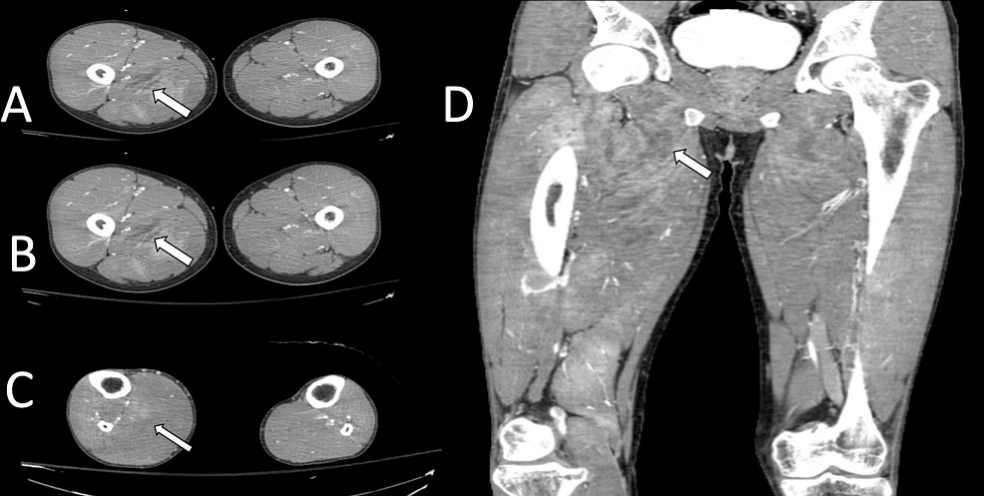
Axial and coronal computed tomography images of the lower extremities Axial and coronal computed tomography images of the lower extremities show extensive and diffuse myositis (hypointense signal) (axial A–C, coronal D)
